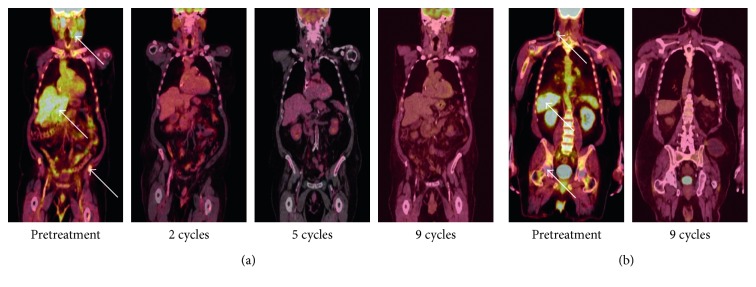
Staging imaging demonstrating response to treatment. (a) PET/CT imaging at initiation of treatment, after 2 cycles of treatment, 5 cycles of treatment, or 9 cycles of treatment as listed. (b) PET/CT imaging showing activity in the bone marrow and liver prior to treatment that was reduced to background levels after 9 cycles of treatment. White arrows indicate areas of active disease on the PET/CT.
